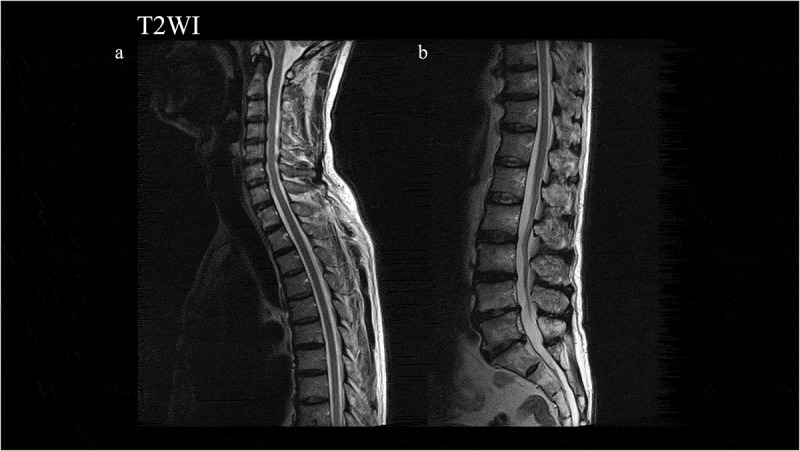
Magnetic resonance imaging findings in T2-weighted images (T2WI) in the spinal cord. Normal findings were observed.
